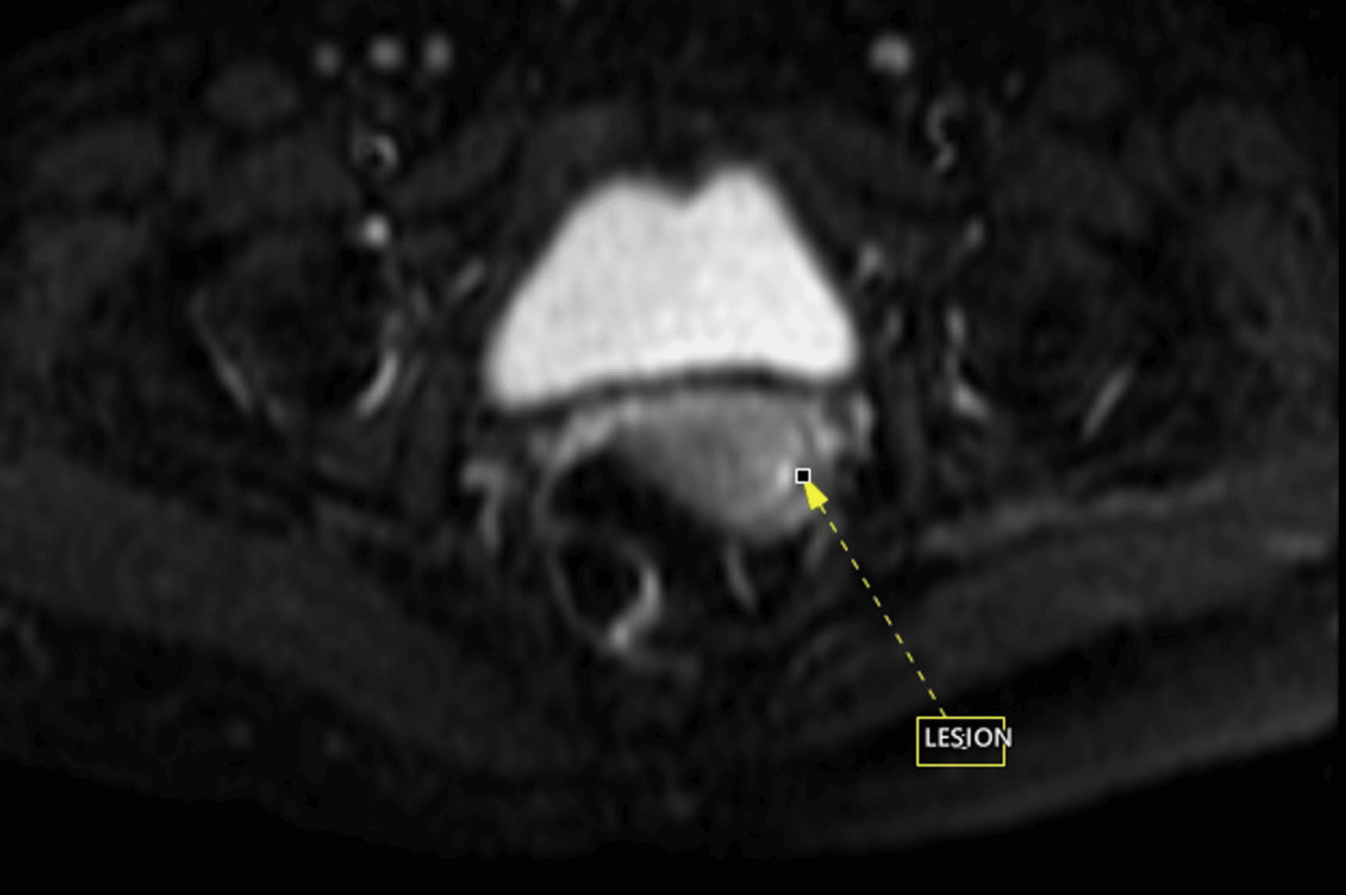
Diffusion-weighted imaging (DWI) sequence magnetic resonance imaging (MRI) at the level of the vagina shows lesion in the vaginal vault showing diffusion restriction
